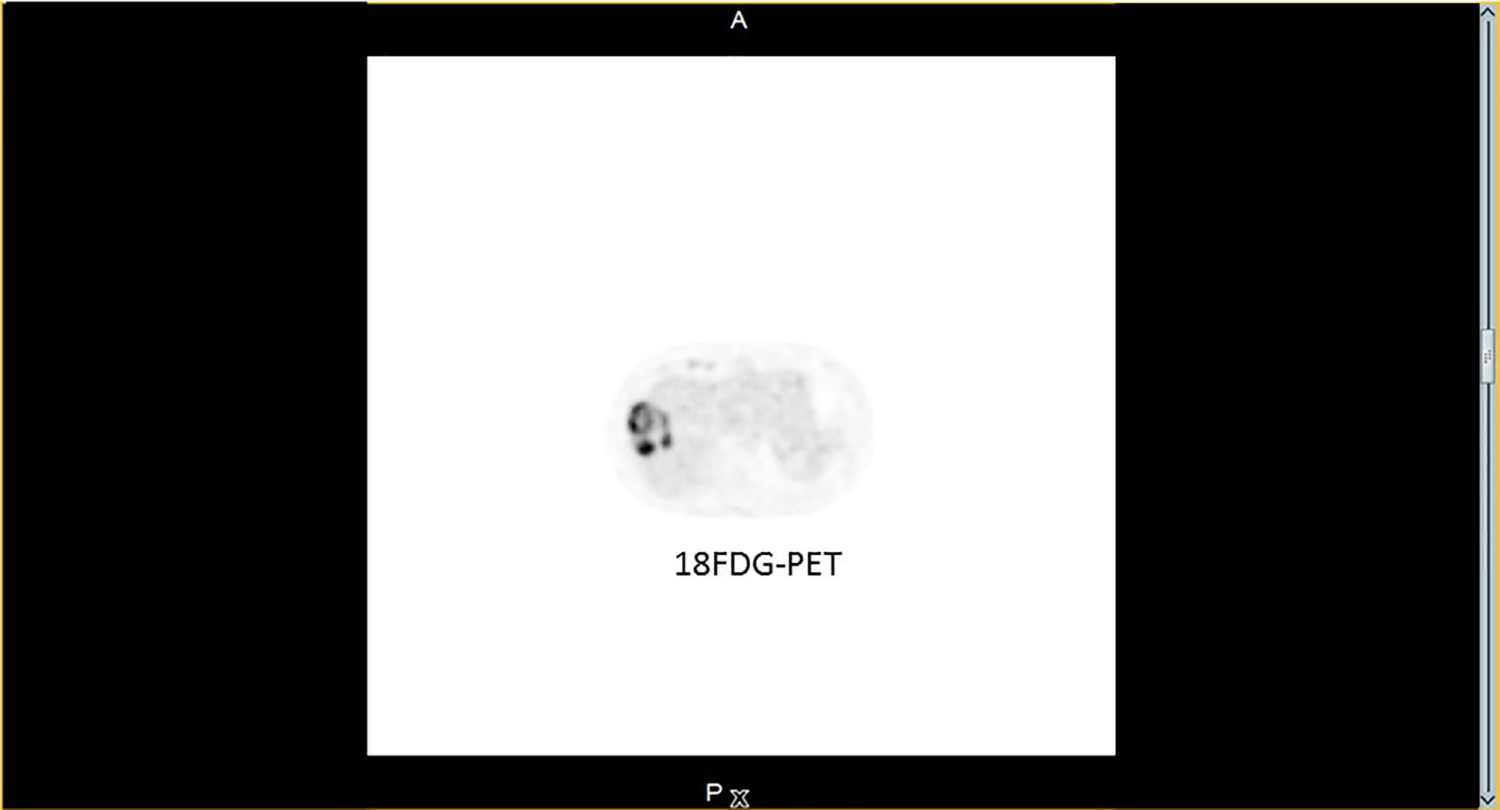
^18^FDG-PET. ^18^FDG-PET image from March 2016: major uptake in the progressing lesion; no other suspect foci was seen.
